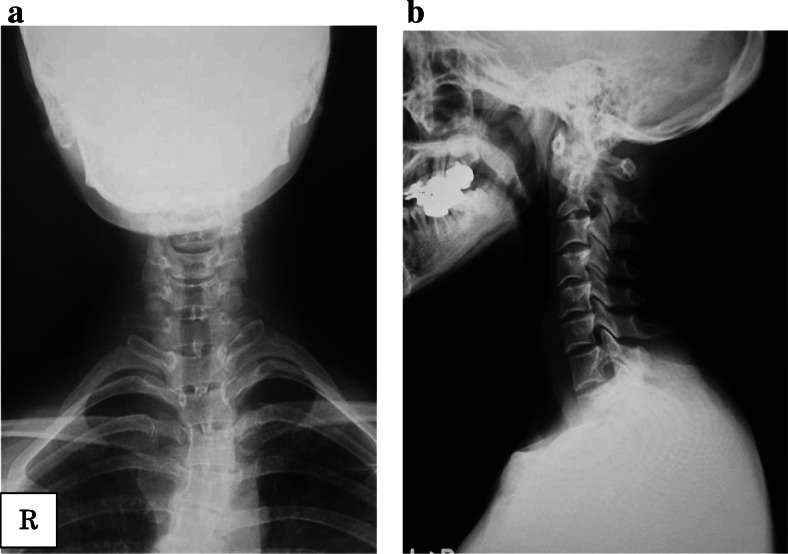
First operation. a Anterior–posterior radiography image at the first operation. b Sagittal radiography imaging at the first operation. The taping system and the iliac bone were used for fixation between C1 and C2. The upper atlantodental interval was 3.5 mm
